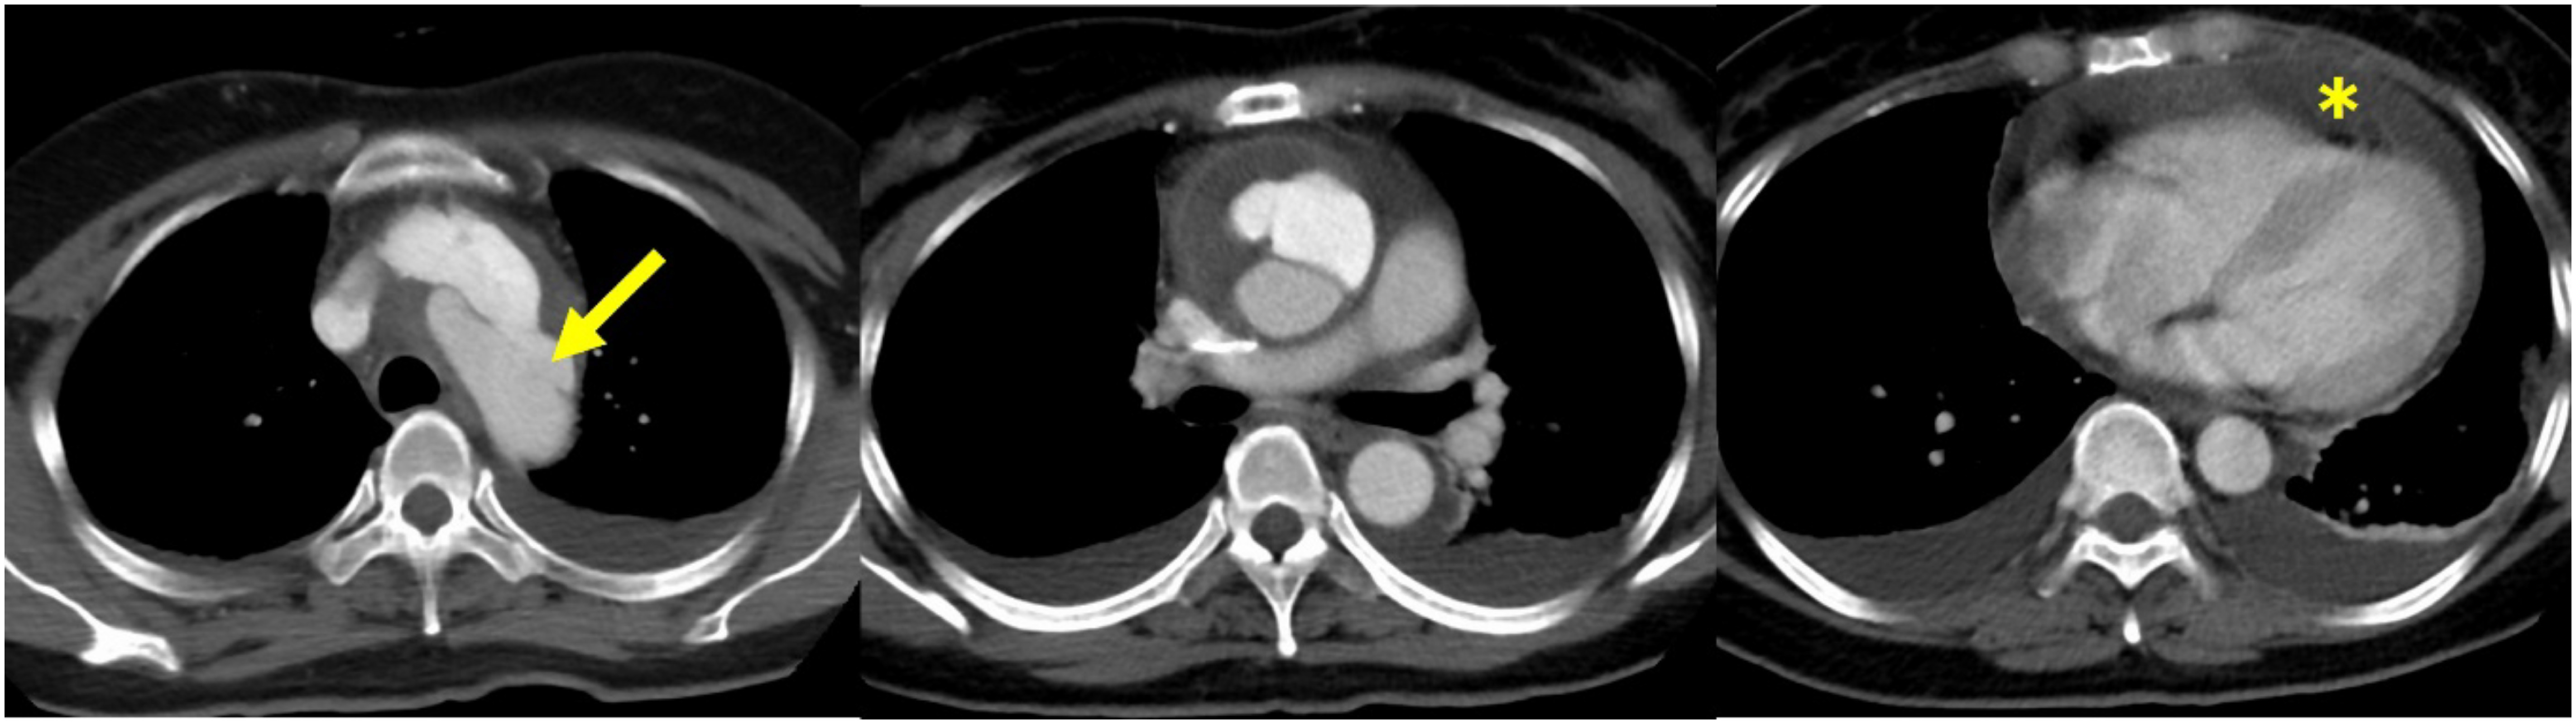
Fig. 1 Contrast-enhanced computed tomography image showing type A acute aortic dissection with patent false lumen with a maximum diameter of 32 mm, intimal tear in the aortic arch (arrow), and moderate pericardial effusion (asterisk). The diameter of dissecting aortic aneurysm of the ascending aorta was 53 mm in a maximum minor axis.
